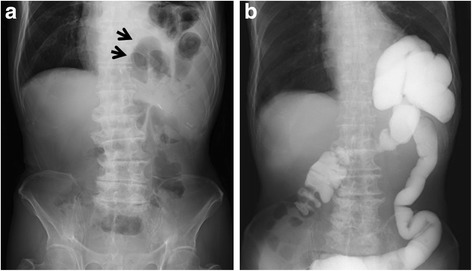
Abdominal X-ray and barium study. a Abdominal X-ray revealed colon gas in the intrathoracic space. b Barium enema examination revealed that the transverse colon was incarcerated in the intrathoracic space
